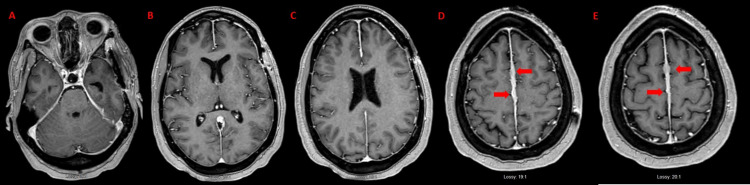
Six-month follow-up MRI post-debulking and RT. MRI post-T1 axial series show (A) overall stable disease at the sella, cavernous sinus, L optic nerve, tentorium, and (B and C) inferior falx. (D and E) Along the superior falx, particularly at the level of the frontal lobes, dural enhancement appears thicker, and more lumpy-bumpy compared to prior. This may signify slow-growing volume of extraaxial disease along the falx (red arrows). RT: radiation therapy; L: left
